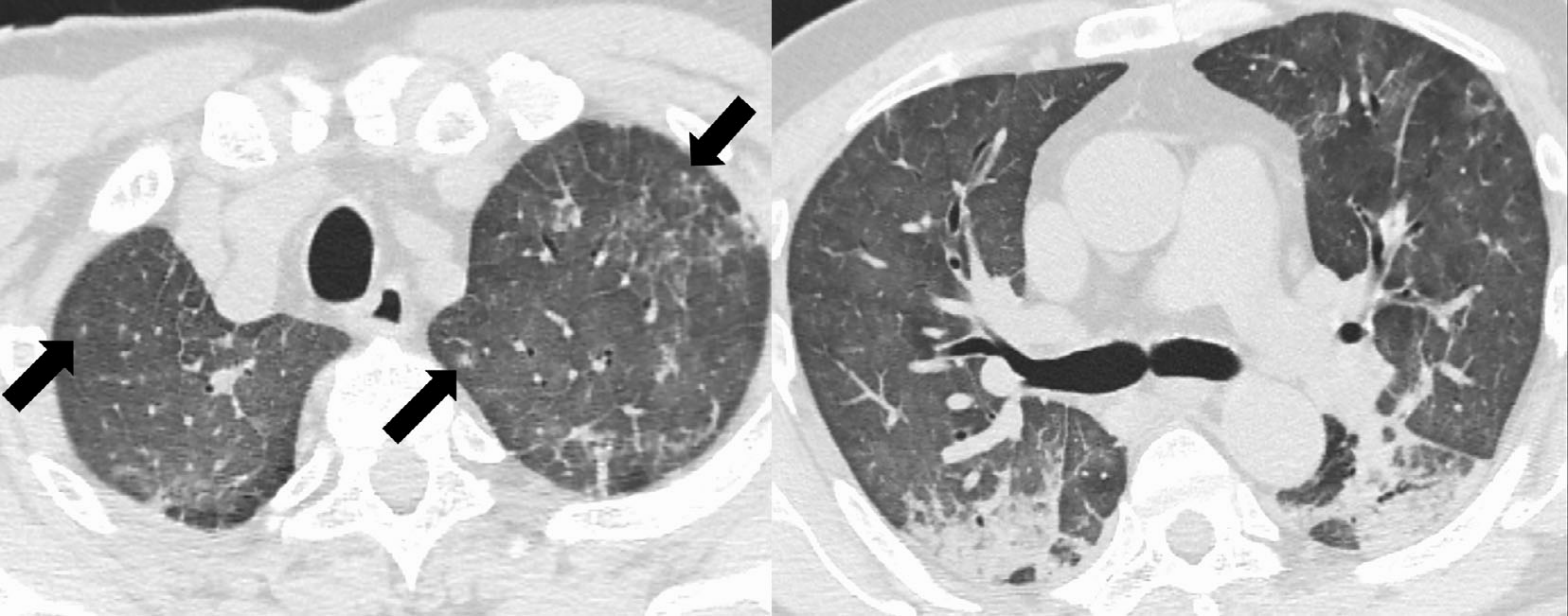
Chest computed tomography (CT) in case 1 showed centrilobular particles (see arrows). Consolidations were seen on both lungs' dorsal area, which was similar to those found in coronavirus disease 2019 (COVID‐19).
